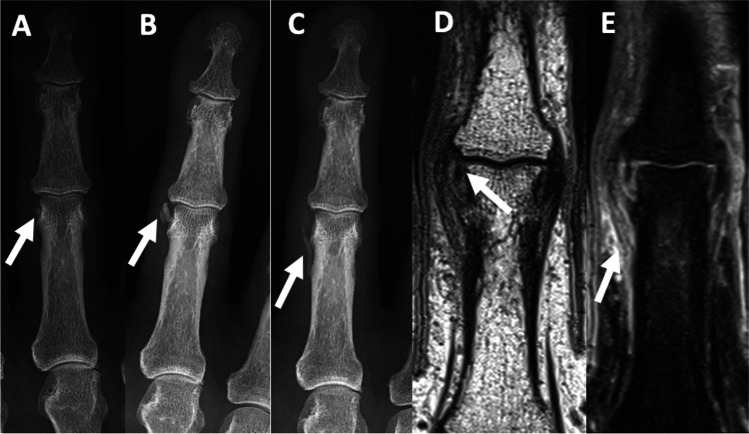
A 77-year-old male. A, B AP radiographs of the finger performed for unrelated wrist pain 3 years apart. The 3rd PIP joint was asymptomatic at this time, however, demonstrating calcification at the radial aspect of the 3rd PIP joint (arrow). The calcification in the later study is larger (arrow). C AP radiograph performed for acute pain involving the radial aspect of the 3rd PIP demonstrates partial resorption of the previously identified calcification and new calcification involving the radial capsule (arrow). D Coronal proton density MRI image demonstrates a small focus of intra-articular calcification at the radial aspect of the PIP joint; however, the capsular calcification is difficult to visualise on MRI. E Coronal proton density with fat-saturated image shows ligamentous/periligamentous edema involving the radial collateral ligament and capsular/pericapsular edema involving the radial joint capsule (arrow)
